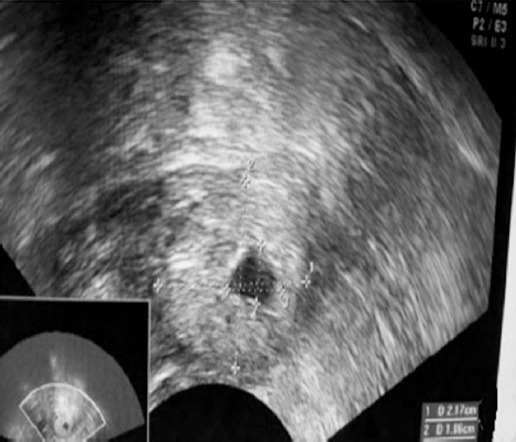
Results of transvaginal sonography: ectopic gestation mass in adnexa area; no obvious gestation sac echo; fuzzy boundary with ovary.
